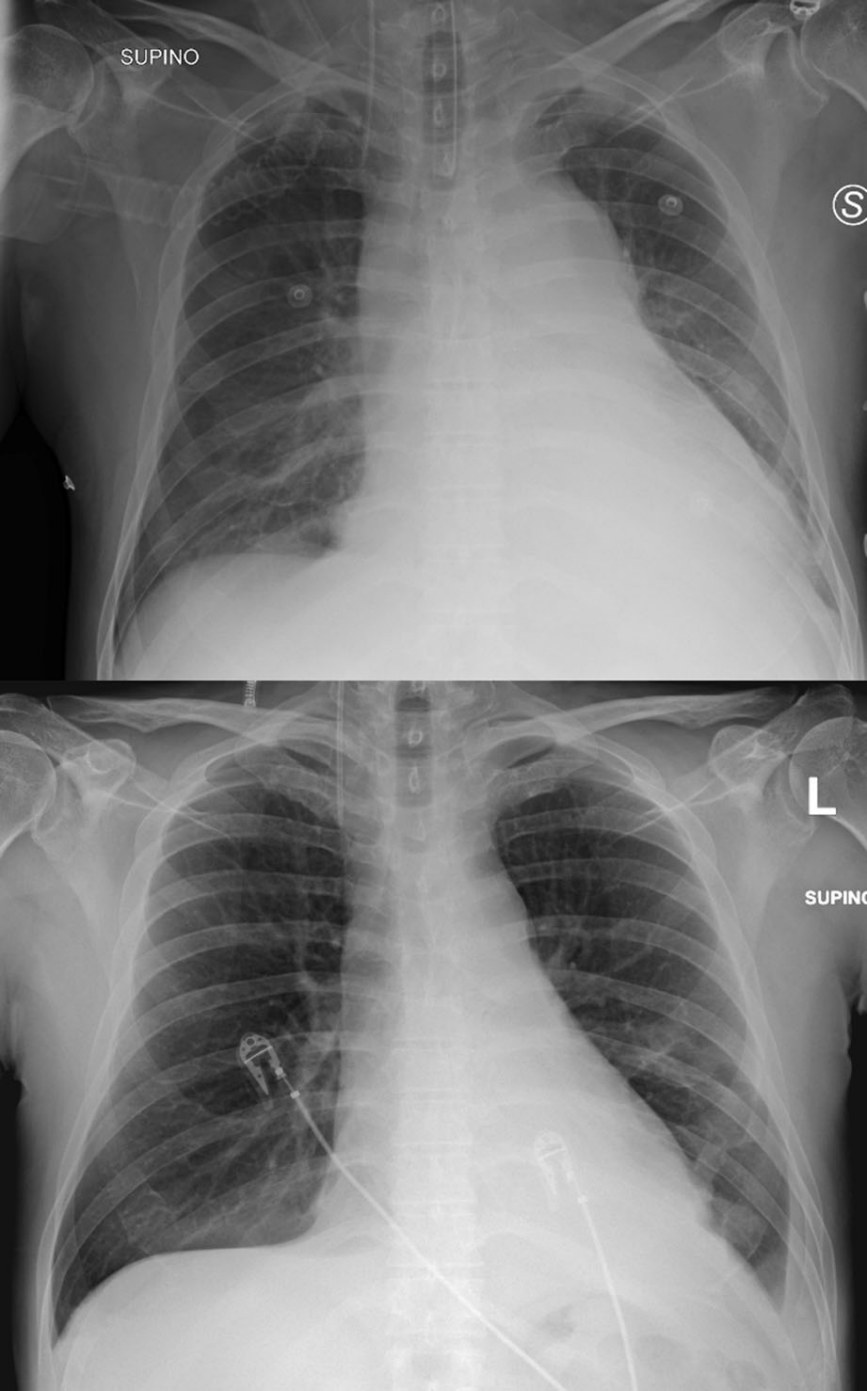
Chest X-ray - The upper picture shows the parenchymal thickening of the left inferior pulmonary lobe with associated pleural effusion. Note the enlarged mediastinum and the increased dimension of the heart. The inferior picture shows the chest X-ray after the first cycle of chemotherapy, with net reduction of the pleural effusion and the mediastinal mass
